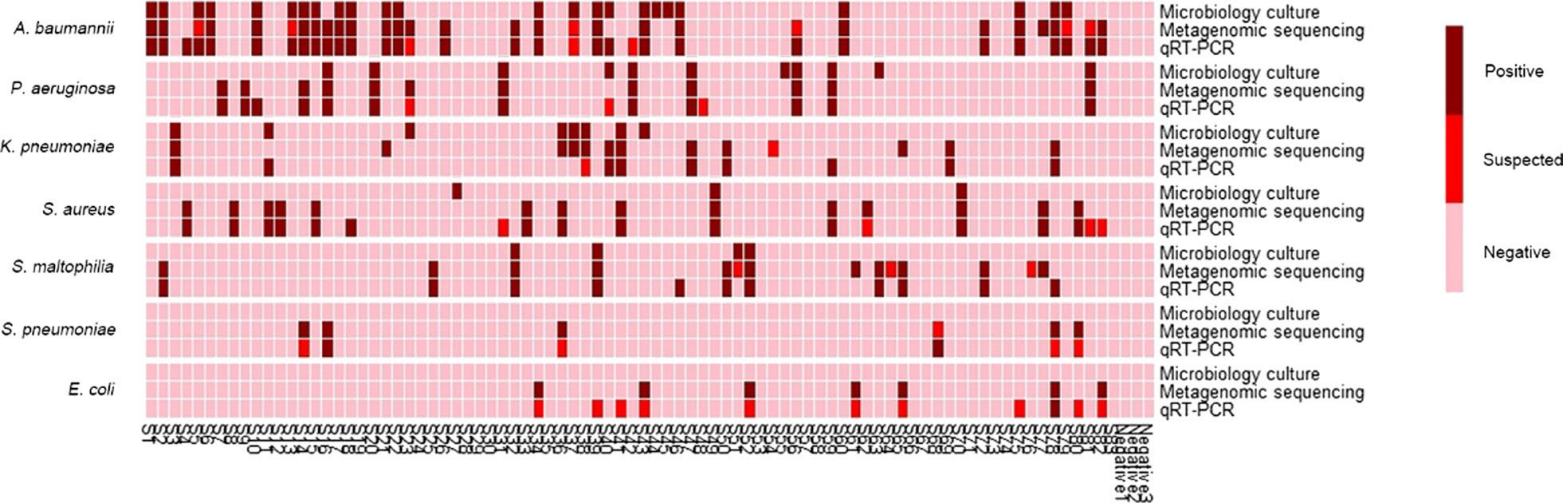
Comparison of microbiological culture, metagenomic sequencing and qRT-PCR. Positive: 1). Microbiology culture: microbiology results report positive; 2). Metagenomic sequencing: reads count over 1 and 1% of all pathogen genomes; 3). qRT-PCR: CT value < 30 and significantly less than negative control; Suspected: 1). Metagenomic sequencing: only has 1 read but over 10% of all genomes; 2). qRT-PCR: CT value between 30–35 and significantly less than negative control; Negative: others. Meeting one of the following conditions indicates that pathogens exist: 1). Microbiology culture positive; 2). qRT-PCR positive; 3). qRT-PCR suspected with metagenomic sequencing positive or suspected
